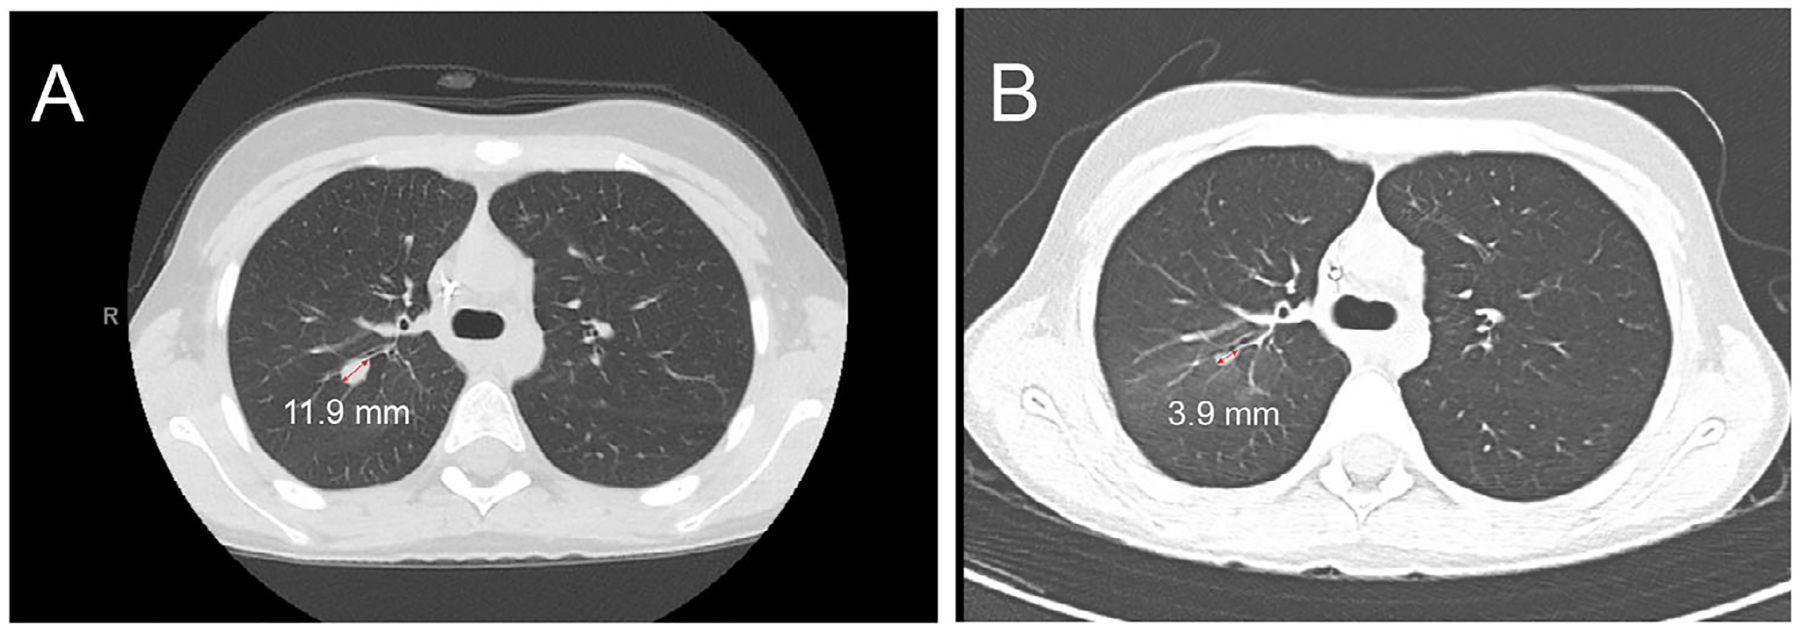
Serial CT chest images of patient 9832–2 with MYOD1-mutant spindle cell/sclerosing RMS who had a sustained PR to VITAS therapy through 16 cycles. Imaging at baseline (A) and after two cycles of protocol therapy (B). CT, computed tomography; RMS, rhabdomyosarcoma; PR, partial response.
